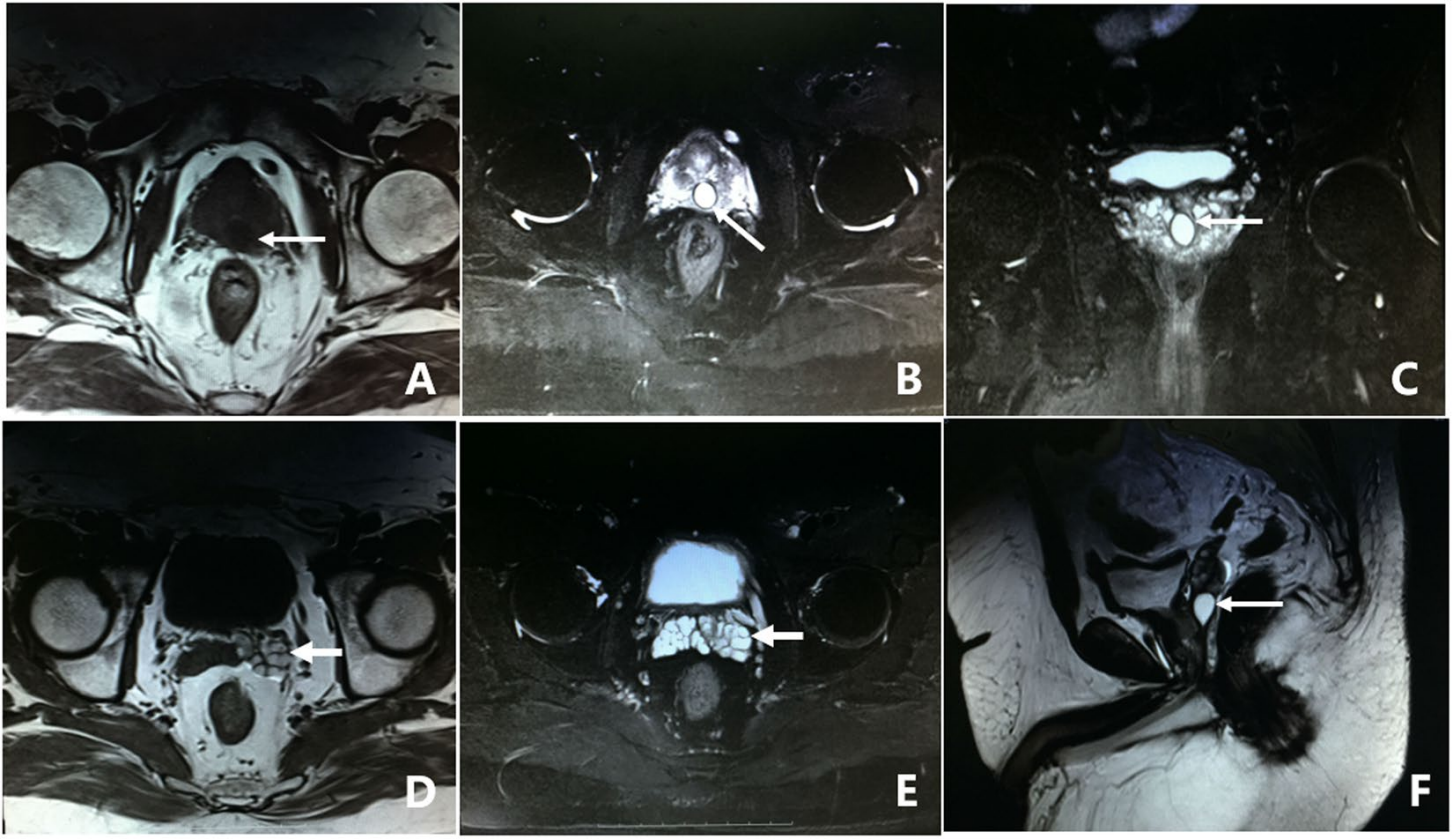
MRI image of PUC. (A) There is a 1.0 × 1.2 cm low-intensity signal cyst in the midline of the prostate on T1WI. (B,C,F) The cyst presents as high-intensity signal on T2WI. (D,E) The left SV presents as high signal intensity on both T1WI and T2WI, and its width has expanded to 2.1 cm. This pattern suggests that the cyst is a PUC and is accompanied by enlargement and an old hemorrhage in the left SV.
